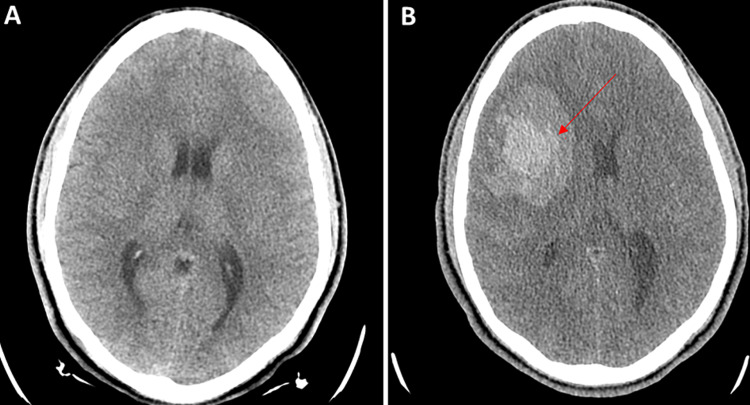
(A) Axial cut, non-contrast head CT on admission was unremarkable. (B) Axial cut, non-contrast head CT four hours after admission demonstrated 6.4 x 4.4 x 4 cm right fronto-parietal intracranial hemorrhage with mass effect and 7 mm right to left midline shift. Subarachnoid hemorrhage in the right frontal and temporal lobes.
